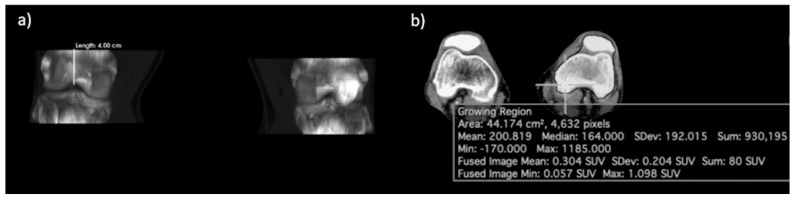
Delineation of the region of interest (ROI) of the knee joint. (a) Coronal view of the CT segmentation showing the boundary 4 cm above and below the intercondylar eminence. (b) Axial view showing the process of morphological closing.
